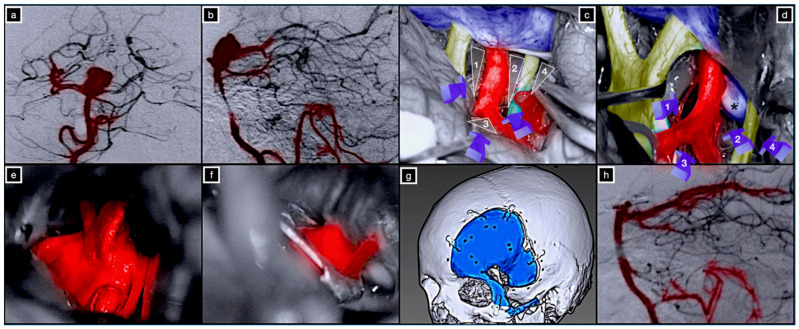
Preoperative digital subtraction angiography of the right vertebral artery in anterior–posterior (a) and lateral (b) projection. (c,d) Intraoperative pictures showing the right optic-carotid (1), carotid-oculomotor (2), supracarotid (3), and oculomotor-tentorial (4) deep windows * posterior clinoid process. Exposure (e) and clip ligation (f) of the basilar tip aneurysm. (g) Three-dimensional (3D) volume-rendered postoperative CT scan highlighting the COZ approach. (h) Postoperative digital subtraction angiography of the right vertebral artery in lateral projection.
